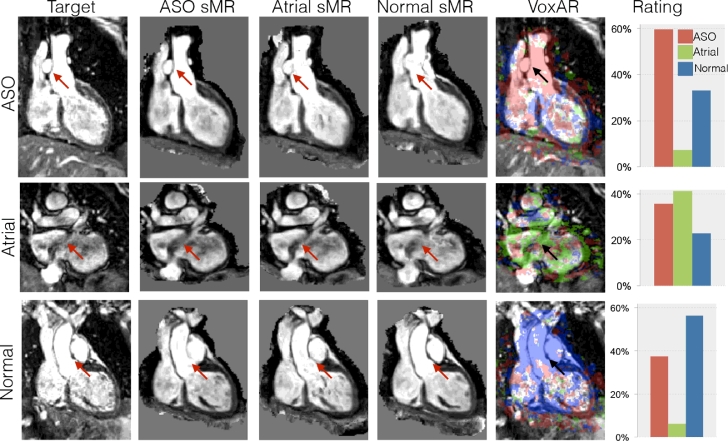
Diagnosis examples. For every target (first column), synthetic MR images (sMR) are obtained using three atlas databases, ASO, atrial and normal, with ISA. Arrows point to areas where morphological differences between the atlas databases and the target are prone to produce anatomically inconsistent images. The fourth column displays the target image with the rating map obtained through VoxAR, followed by the corresponding rating histogram.
